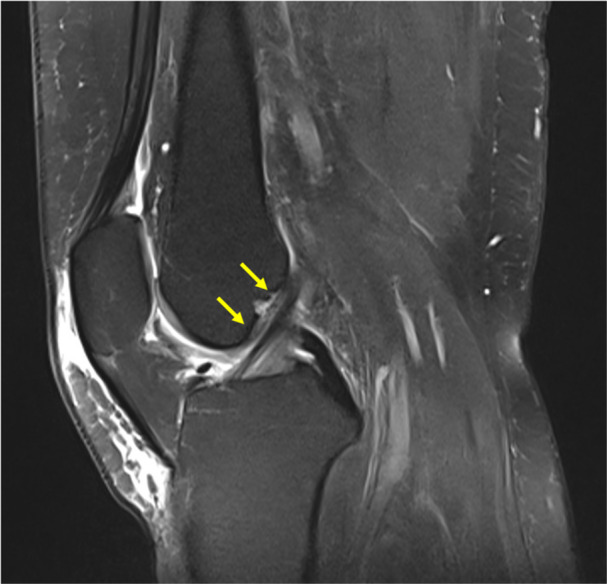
Sagittal fat‐suppressed T2‐weighted MR image of a patient with intact cruciate ligaments. The ACL runs parallel to the ‘Blumensaat line’ and maintains close contact with the roof of the intercondylar notch (→). ACL, anterior cruciate ligament; MR, magnetic resonance.
